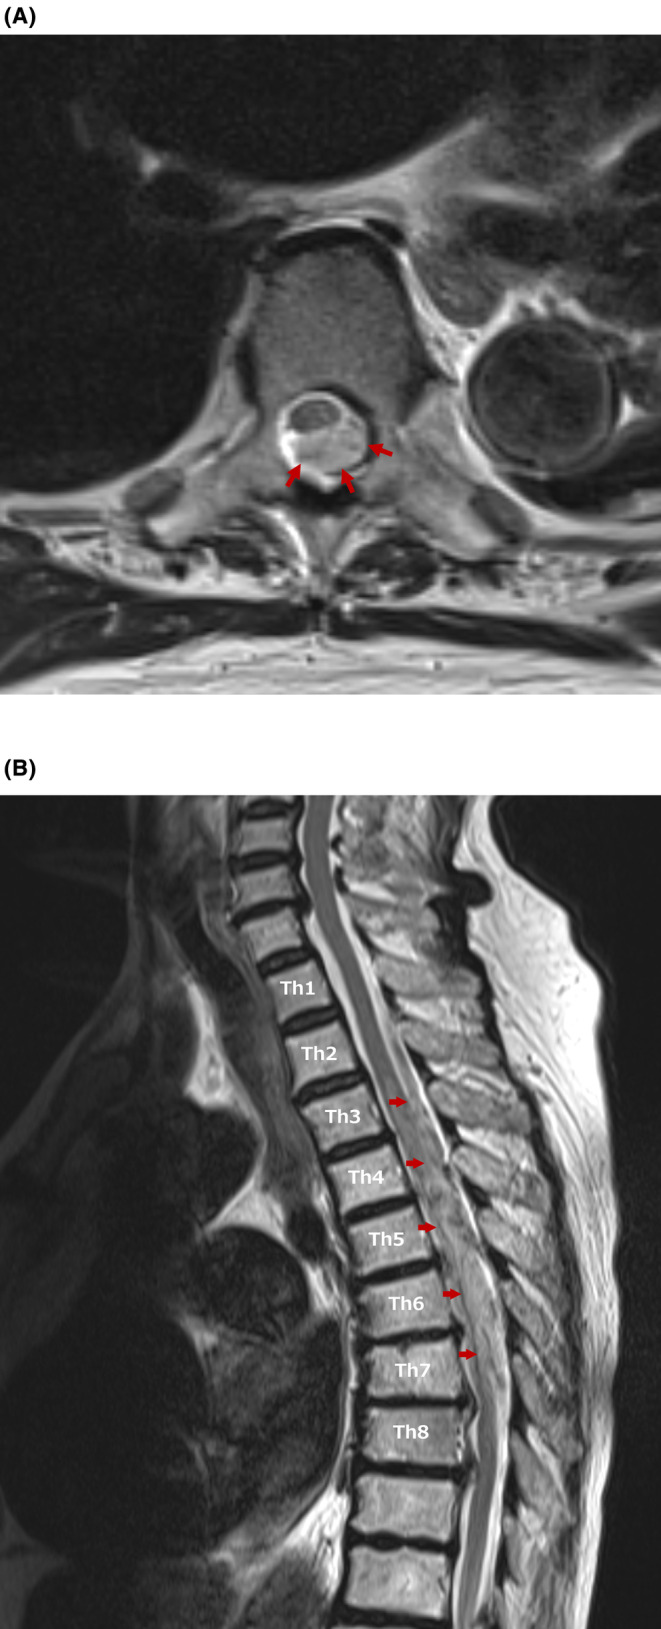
Axial and sagittal magnetic resonance imaging. (A) Axial magnetic resonance imaging of the thoracic spine reveals a spinal epidural hematoma at six thoracic vertebral levels (red arrows). (B) Sagittal magnetic resonance imaging reveals a spinal epidural hematoma from thoracic vertebra 2–7 (red arrows).
